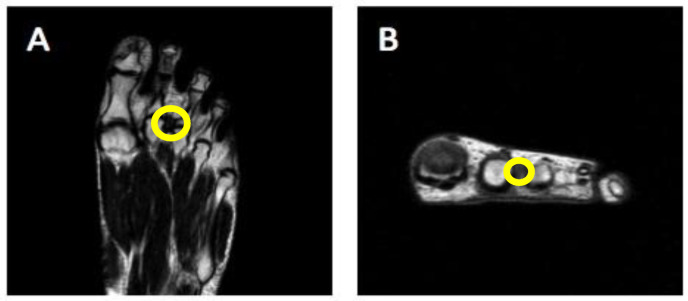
(A,B) MR imaging of Morton’s neuroma, identifiable as a hypointense soft-tissue nodule at the level of the second intermetatarsal space. The presence of MN is indicated by a yellow circle.
